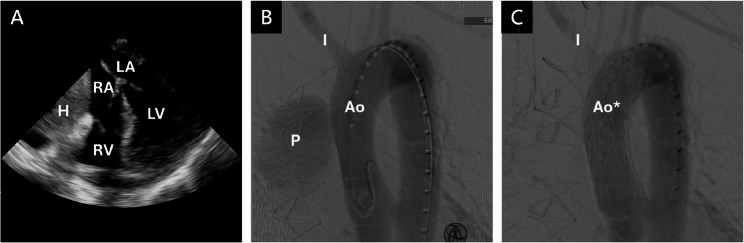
Transesophageal Echocardiography and Angiography Images of the Aortic Pseudoaneurysm. (A) TEE showed that the right atrium and right ventricles were compressed by the huge hematoma. (B, C) Angiography before and after TEVAR. *(H*,* Hematoma; RA*,* Right atrium; RV*,* Right ventricle; LA*,* Left atrium; LV*,* Left ventricle; P*,* Pseudoaneurysm; Ao*,* Aorta; Ao,* Aorta with TEVAR; I*,* Innominate artery)*
